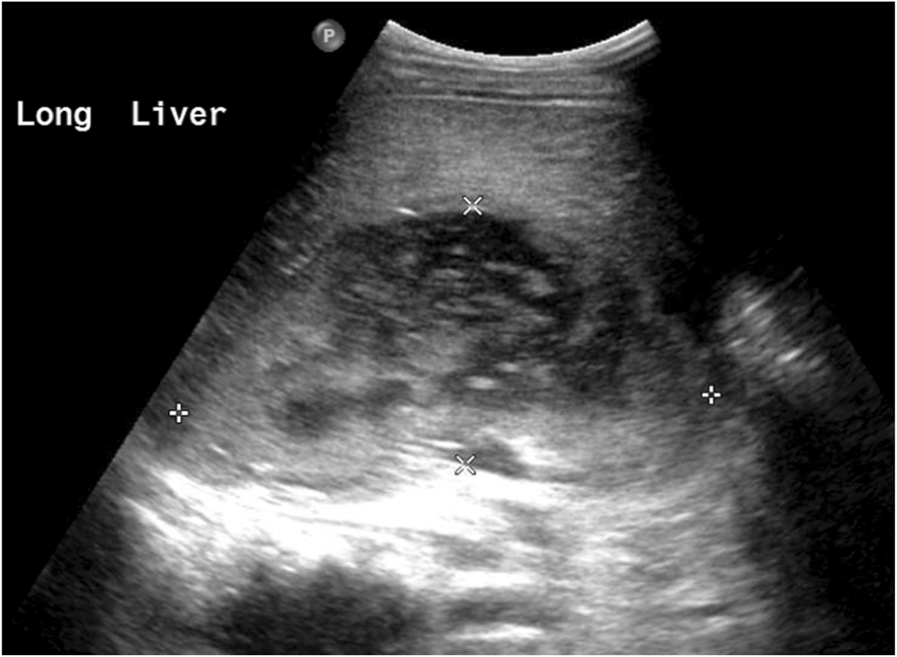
Ultrasound of the abdomen revealing a hypoechoic avascular area within the right upper quadrant. This complex fluid collection appears to have an intimate relationship with the liver parenchyma.
